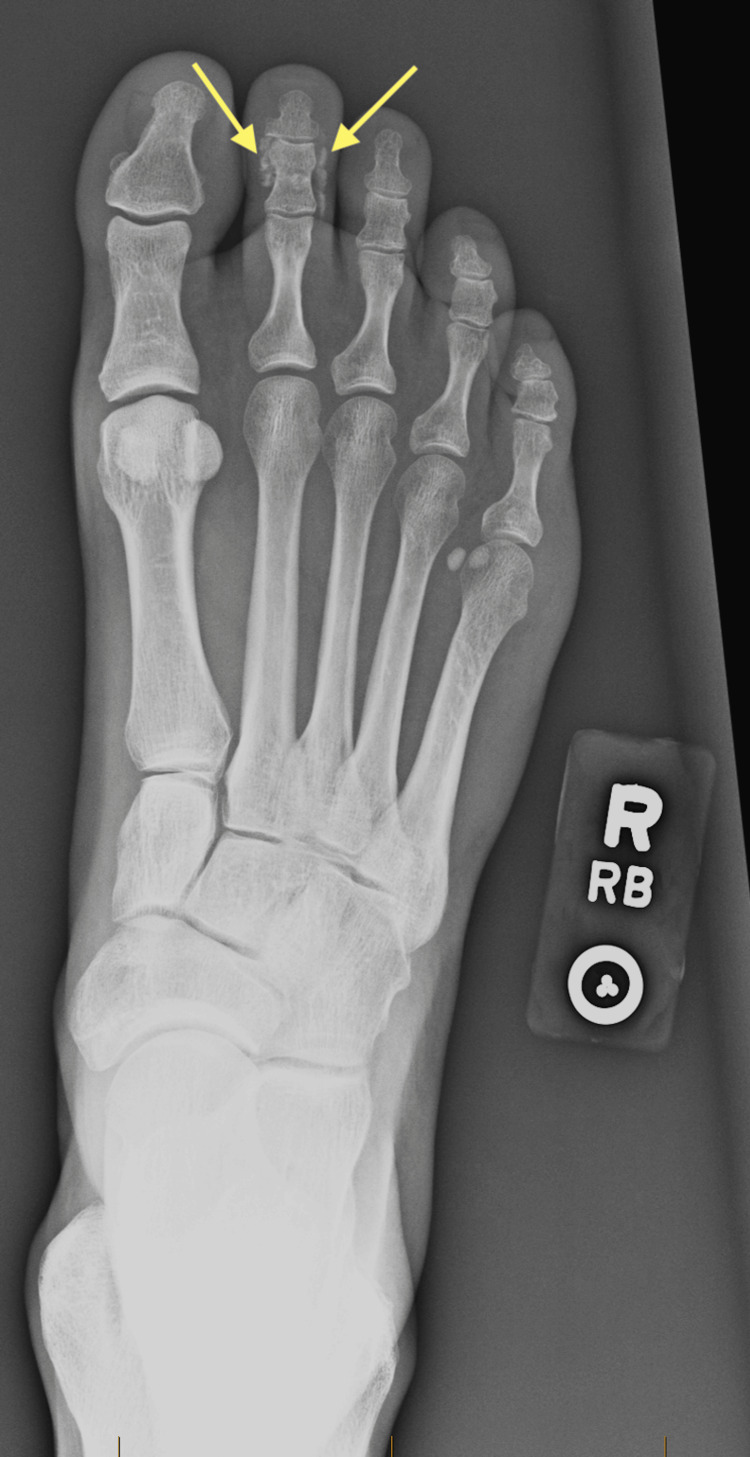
X-ray confirming periarticular calcification (arrows)
